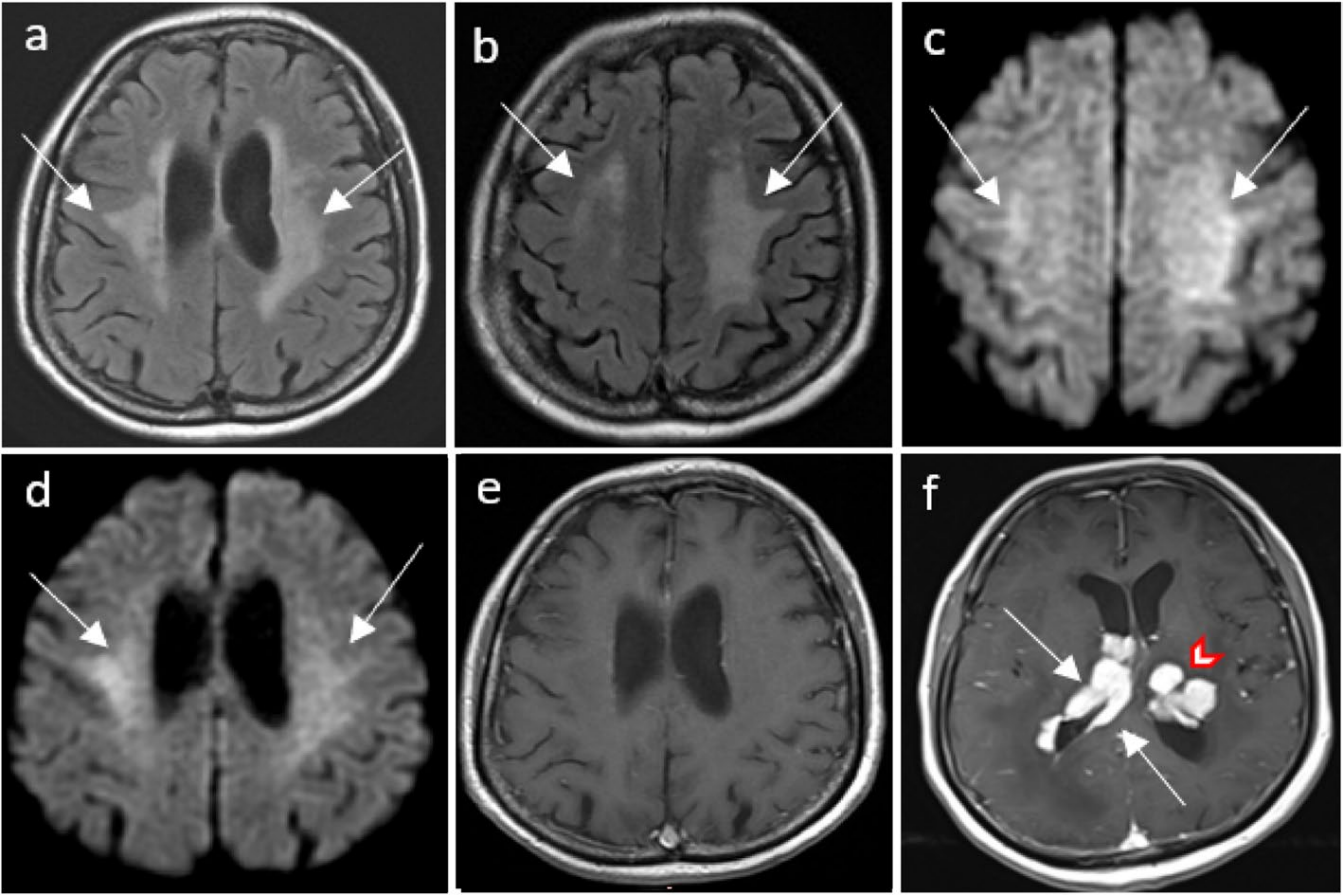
Methotrexate induced encephalopathy. A 64-year-old immunocompetent female has been diagnosed with primary central nervous system (CNS) lymphoma. High dose of methotrexate-based chemotherapy was administered. In the second week of treatment, she complained of bilateral upper limb weakness and numbness, as well as dysphagia. Axial FLAIR images (a, b) show hyperintensities in bilateral centrum semiovale and corona radiata sparing the subcortical U-fibers. Axial diffusion-weighted images (DWI) (c, d) show corresponding increased signal and low apparent diffusion coefficient (ADC) (not shown). The aforementioned areas with FLAIR and diffusion abnormalities do not show any post-contrast enhancement, and they represent methotrexate-induced leukoencephalopathy. Axial contrast-enhanced T1-weighted images (e, f) show enhancing lesions in both lateral ventricles including the ependymal and subependymal regions (arrows, f) as well as the left thalamus (red outlined arrowhead, f) are consistent with lymphomatous lesions
